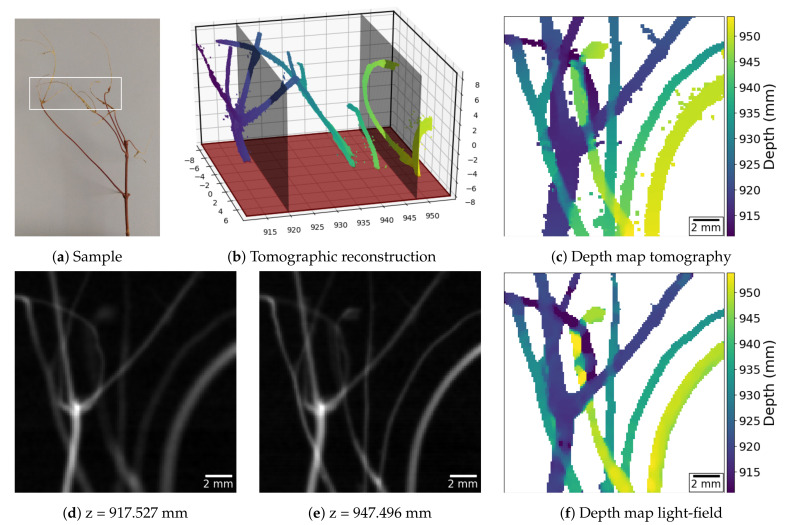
X-ray light-field imaging of a tree branch to demonstrate the ability to capture the three-dimensional (3D) structure of vessel-like objects. The sample is shown in (a), where the white box identifies the analyzed region. The traditional CBCT reconstruction is rendered in (b). (d,e) present the refocus planes shown in (b). (c,f) present the depth maps extracted from the tomographic reconstruction and the light-field acquisition, respectively.
